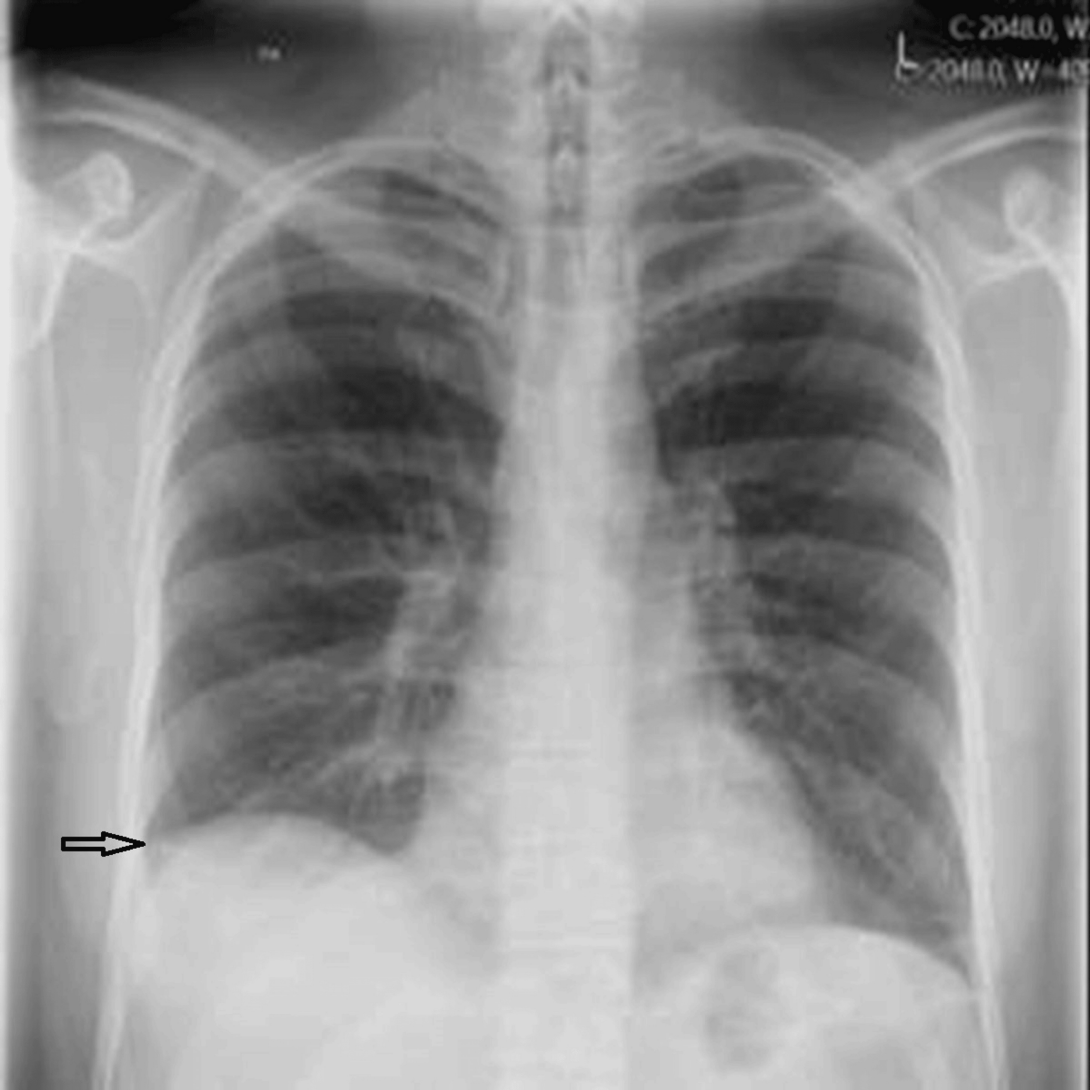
Chest X-ray showing near-complete resolution of left-sided pleural effusion without drainage and minimal right-sided pleural effusion without drainage.
